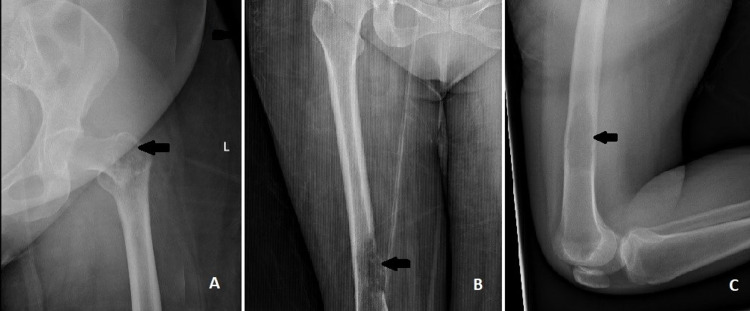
(A) X-ray of the left femur showing intertrochanteric fracture and abnormal, suspicious lesion at the fracture site (arrowhead); (B) and (C) X-ray of the right femur showing osteolytic lesion (arrowhead).
